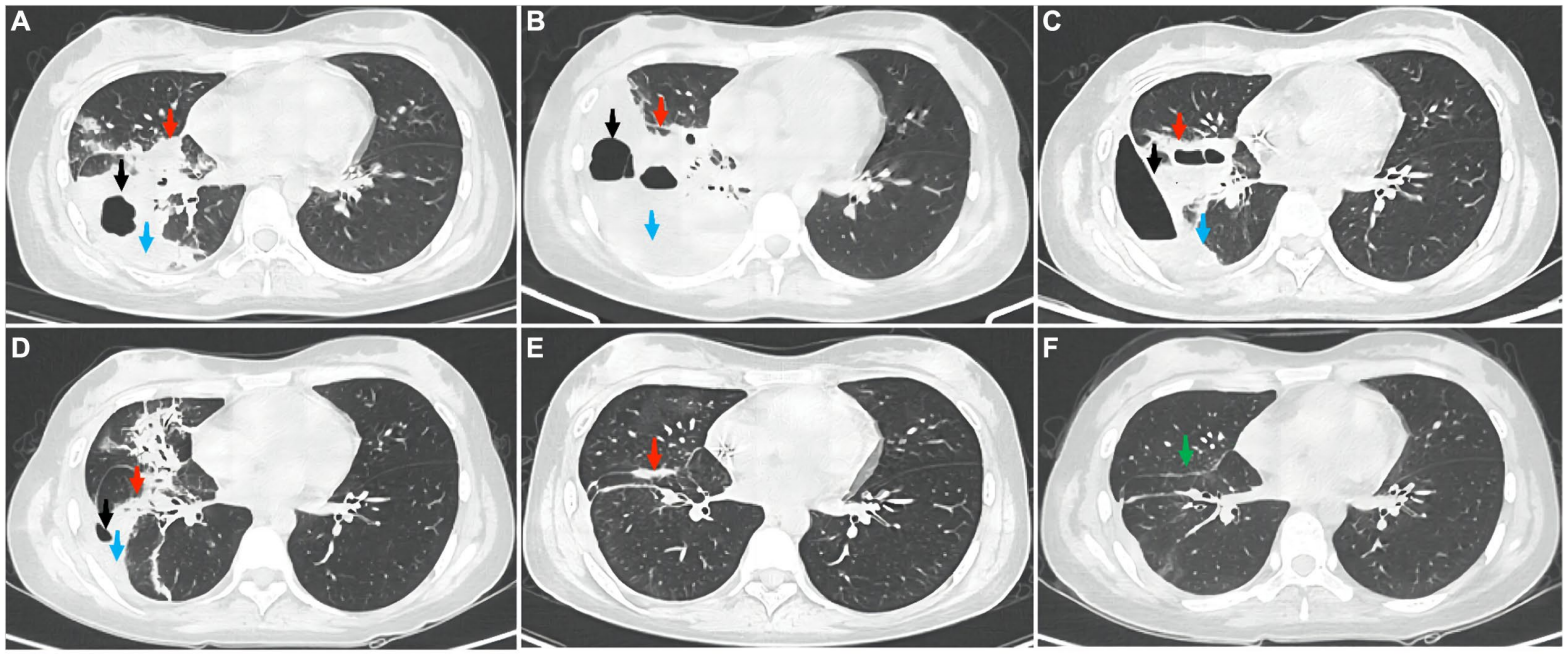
The picture shows the changes in the chest CT images during treatment. (A) the first CT scan in the hospital taken on 29 June 2023. The patient underwent a CT scan of the chest to observe the therapeutic effect on (B) 12 July 2023 and (C) 31 July 2023. Outpatient re-examinations using a repeat CT scan on (D) 25 August 2023, (E) 3 October 2023, and (F) 14 November 2023. The red arrow represents the inflammatory lesion, the black arrow shows the cavity, the blue arrow indicates fluid accumulation, and the green arrow represents residual striped shadows.
